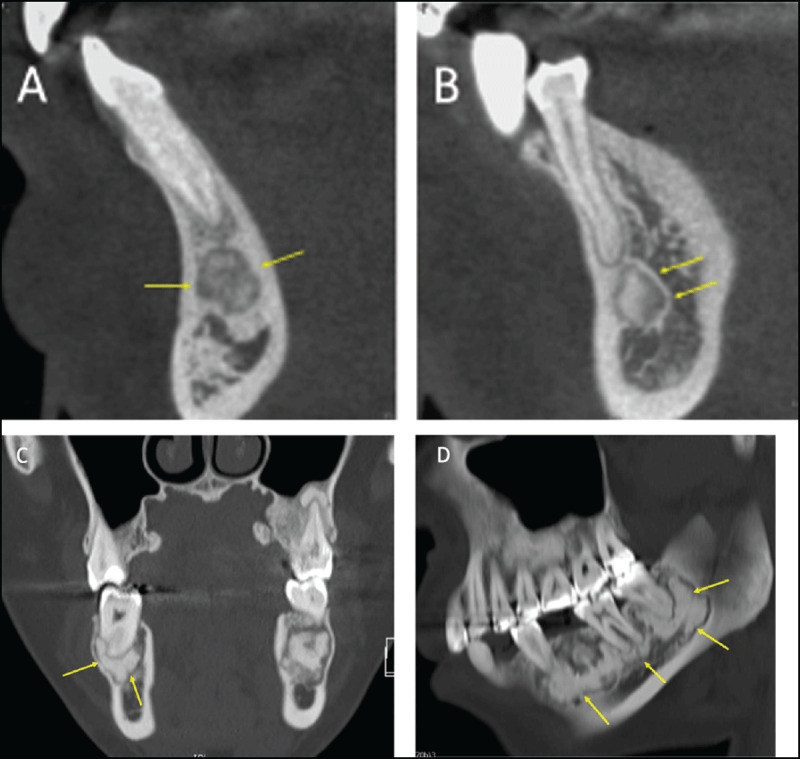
(A) Periapical cemento-osseous dysplasia in two different patients. CT reformations showing periapical COD lesions clearly separated from the root of the anterior tooth. The lesion in (a) has a sclerotic center and a more radiolucent peripheral part (arrows). The lesion in (B) has a “target appearance” with a central sclerotic part, an intermediate more lucent part, and a thin peripheral sclerotic rim (arrows). (C, D) Florid cemento-osseous dysplasia. On CT reformations, multiple sclerotic periapical lesions involve the mandible bilaterally; the lesions have an elliptical shape and are clearly separated from the root of the mandibular teeth (arrows).
